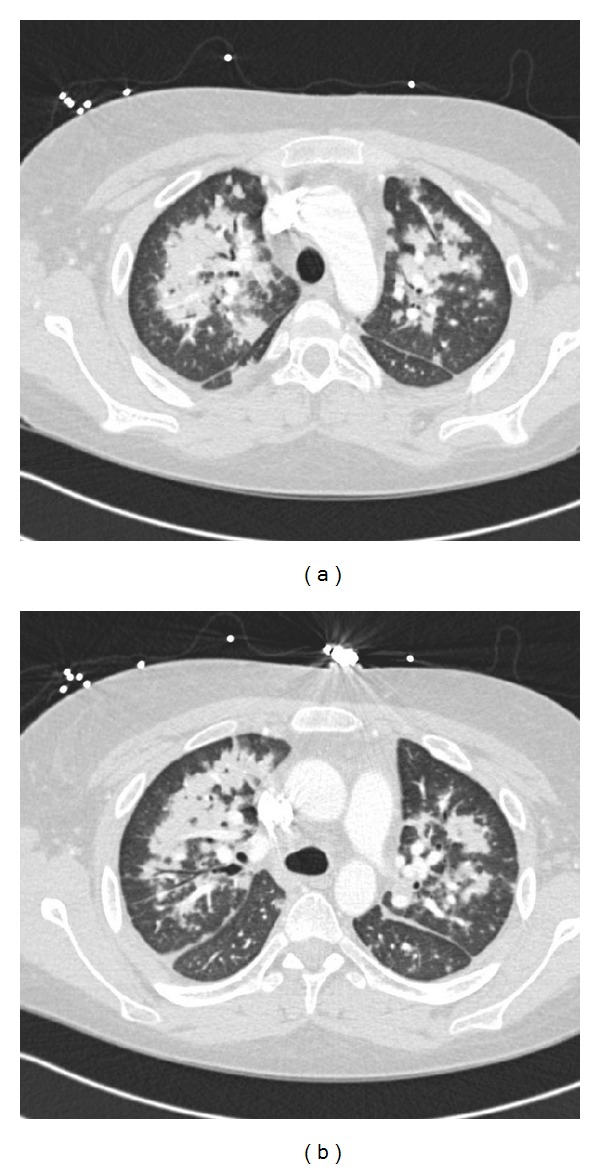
CT Thorax obtained after patient developed hemoptysis and dyspnea showing bilateral alveolar infiltrates.
